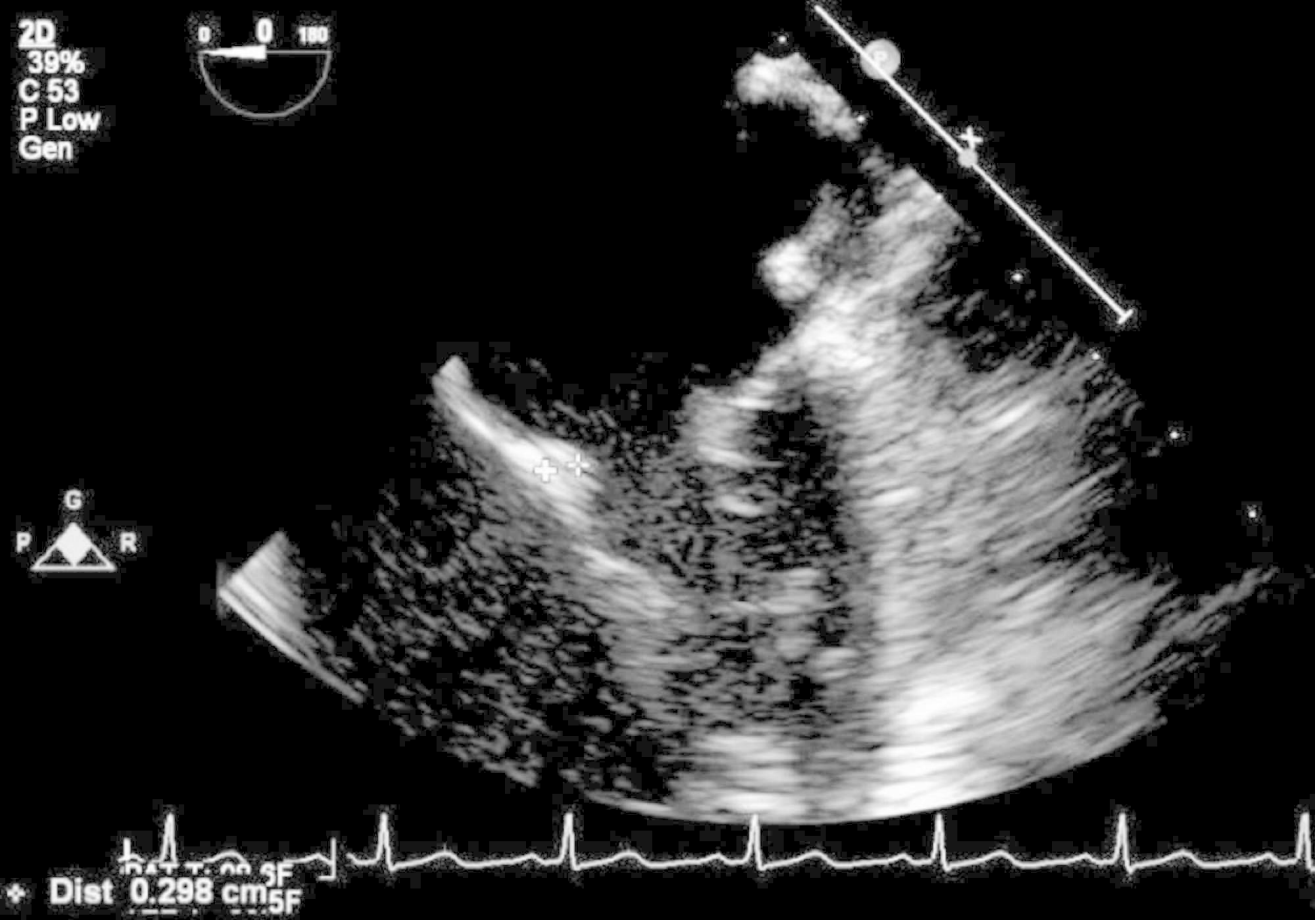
Transesophageal echocardiogram showing 0.298 cm lobule on the anterior leaflet of the mitral valve, indicated by the caliper marks for measuring it, in the lower left portion of the image
